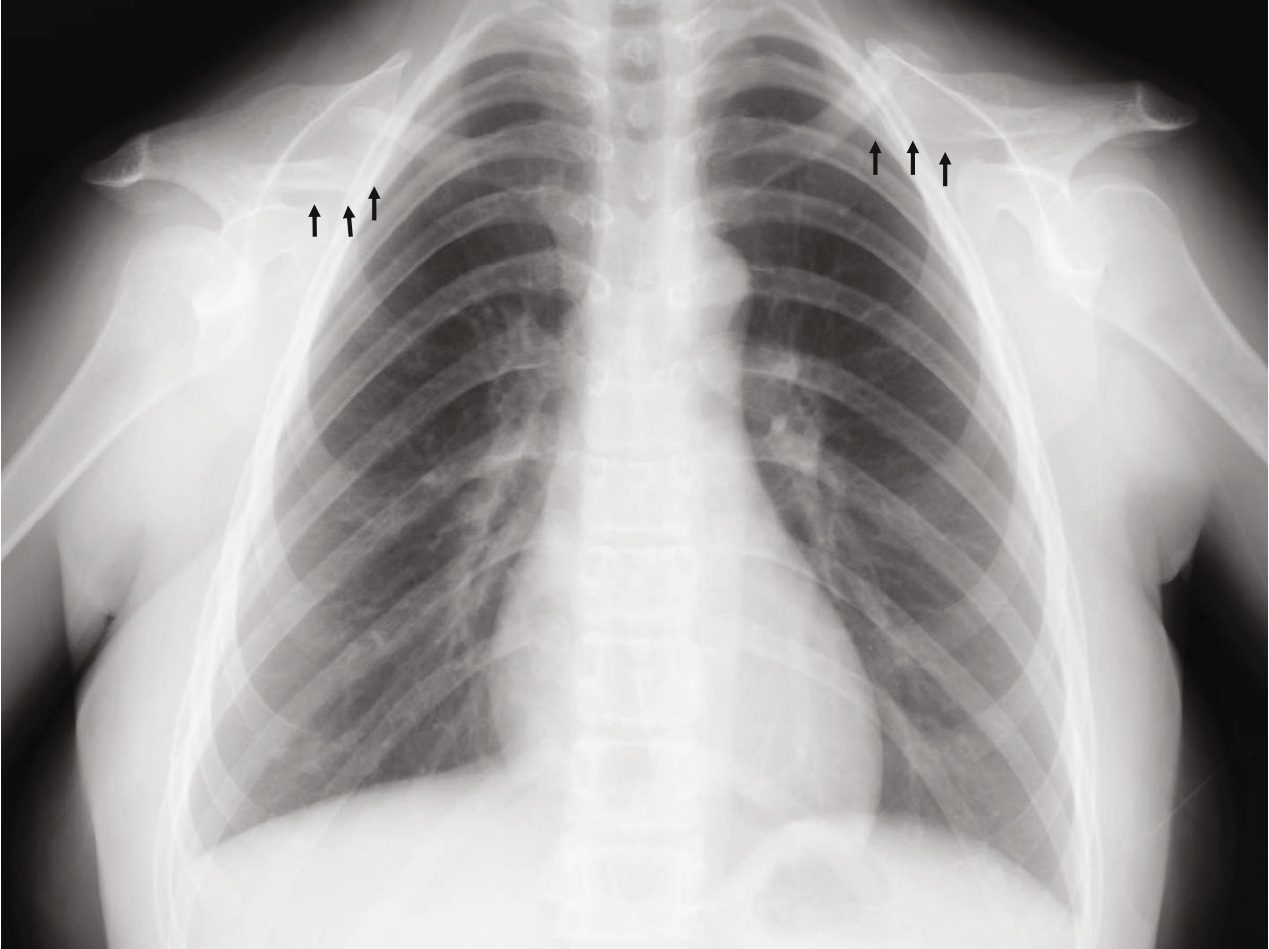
Plain chest radiograph plain chest radiograph (AP view) showing hypoplasia of the clavicles—a characteristic feature of cleidocranial dysplasia (arrows).
